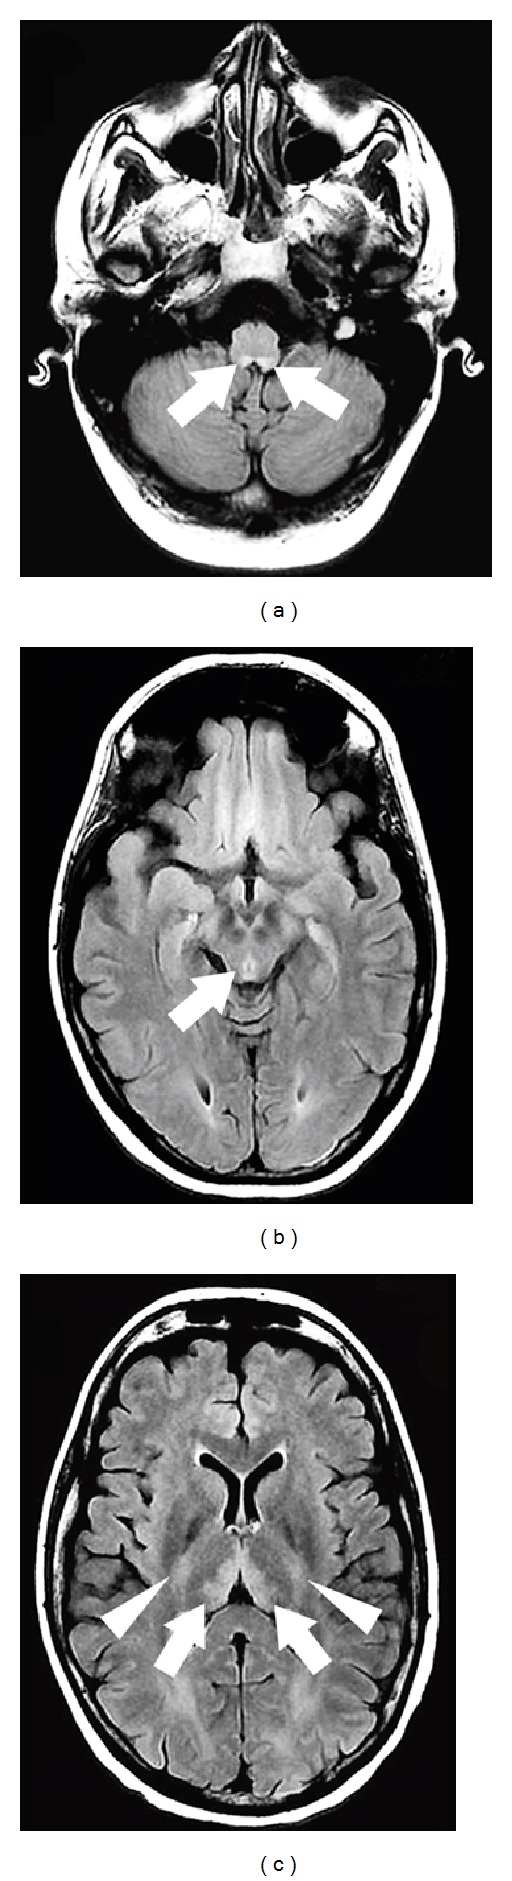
A patient with Wernicke syndrome showing FLAIR hyperintensities in the hypoglossal nuclei (a), the periaqueductal gray matter and mesencephalic tectum (b), the medial part of the thalami (arrows, c), and the pyramidal tracts (arrowheads, c).
